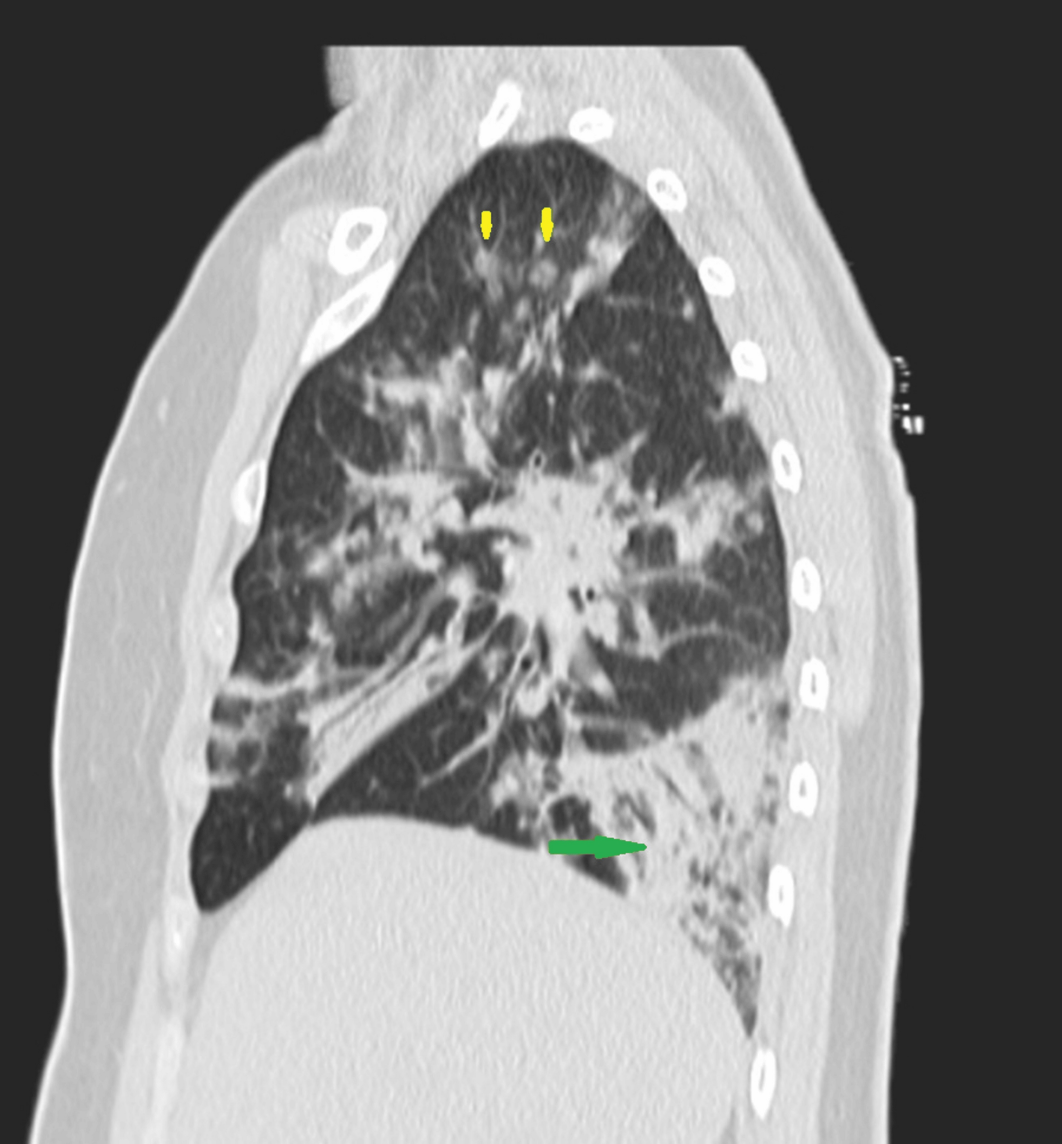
Thoracic CT-scan Green arrow indicates a mosaic/crazy paving pattern; yellow arrows indicate nodular neoplastic lesions
